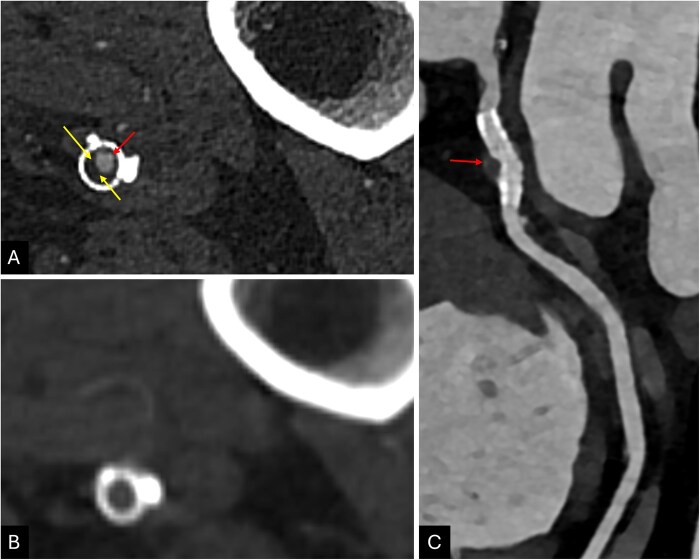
A 64-year-old male with PCD-CTA (A) and EID-CTA (B) runoff study showing a plaque (yellow arrow in A) in a femoral artery stent, stenosing the lumen (red arrow in A), which is completely unseen on EID-CTA. A 78-year-old male underwent PCD-CT coronary angiography (C), showing a moderate stenosis within a circumflex artery stent (red arrow in C). Abbreviations: CTA, CT angiography; EID, energy-integrating detector; PCD, photon-counting detector.
